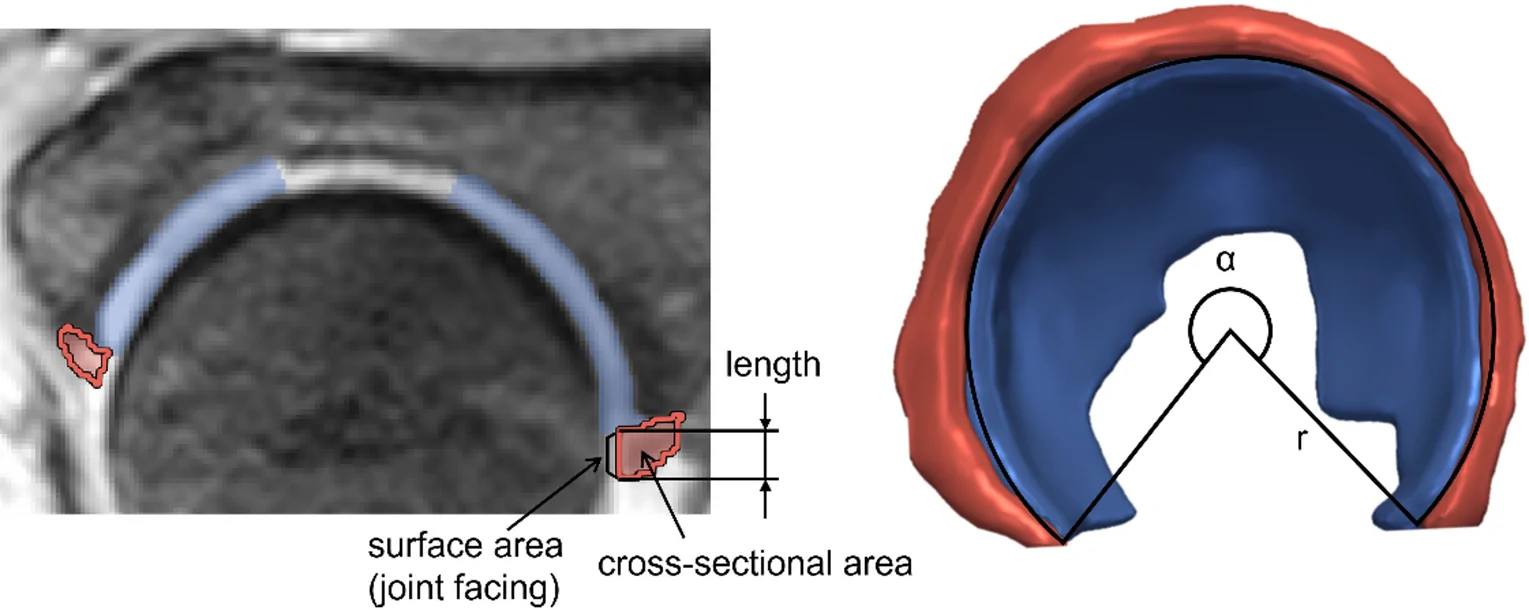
Chondrolabral complex geometric definitions for metric computation. Left: MRI slice with segmented cartilage (blue) and labrum (red). Right: 3D reconstructions. A best-fit sphere to the femoral head provides the radius (* r*); the labral clockface coverage is the angle (α). The labral arc length is $$\:L=\alpha\:r$$, used to compute the average labral cross-section $$\:{V}_{labrum}/L$$ and average labral length $$\:{A}_{labrum}/L$$.
